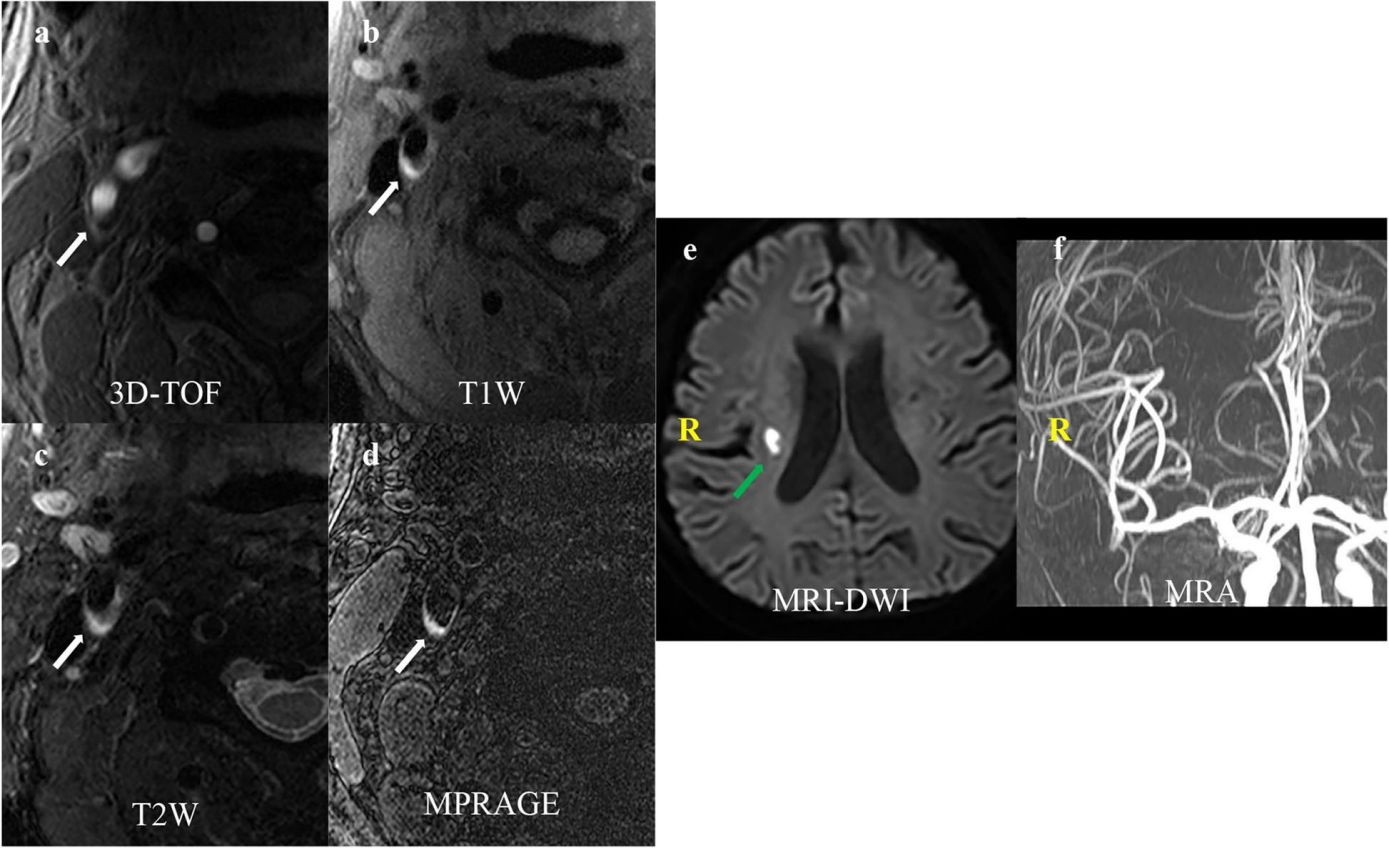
A 64-year-old male patient presented with a right ischemic stroke. IPH is demonstrated by the hyperintensity on (a) 3D-TOF, (b) T1W, (d) MPRAGE, and hyperintensity on (c) T2W (white arrow). There is a hyperintensity lesion in the blood-supply area of the right carotid artery on (d) DWI (green arrow) and no evident ipsilateral intracranial vascular stenosis or occlusion on (e) MRA. DWI, diffusion-weighted images; MRA, magnetic resonance artery; 3D-TOF, three-dimensional time of flight; T1W, T1-weighted; T2W, T2-weighted; MPRAGE, magnetization-prepared rapid acquisition gradient-echo
